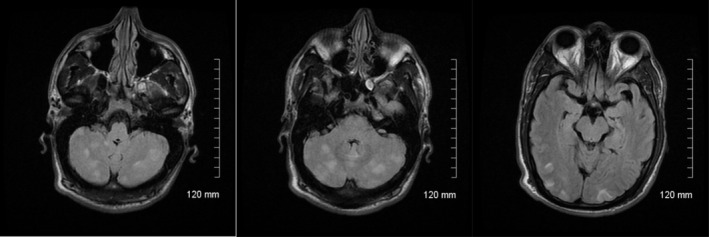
MRI FLAIR sequence on day 2
